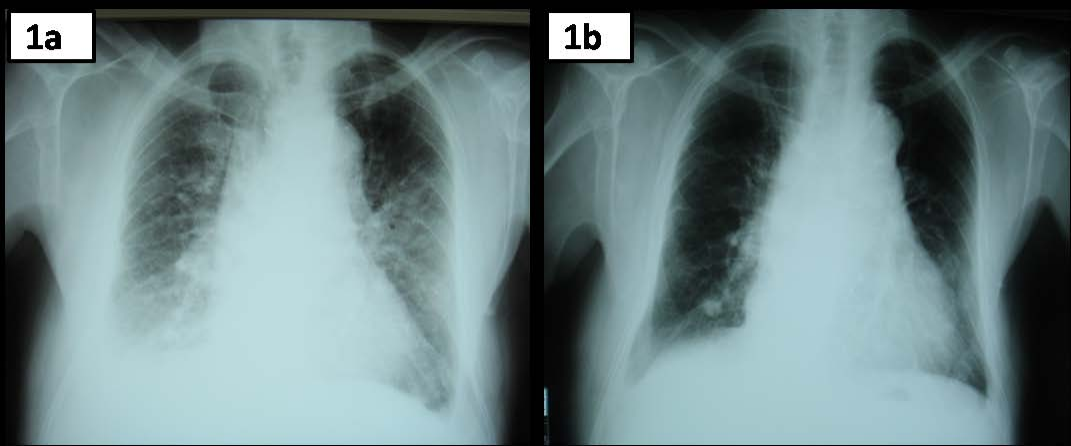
Chest Radiograms. a. Chest X-ray demonstrating bilateral perihilar alveolar edema with a "butterfly" appearance and bilateral pleural effusions. b. Chest X-ray after pharmaceutical treatment for the congestive heart failure symptoms. Most of the initially appeared radiographic findings have been almost completely resolved.
